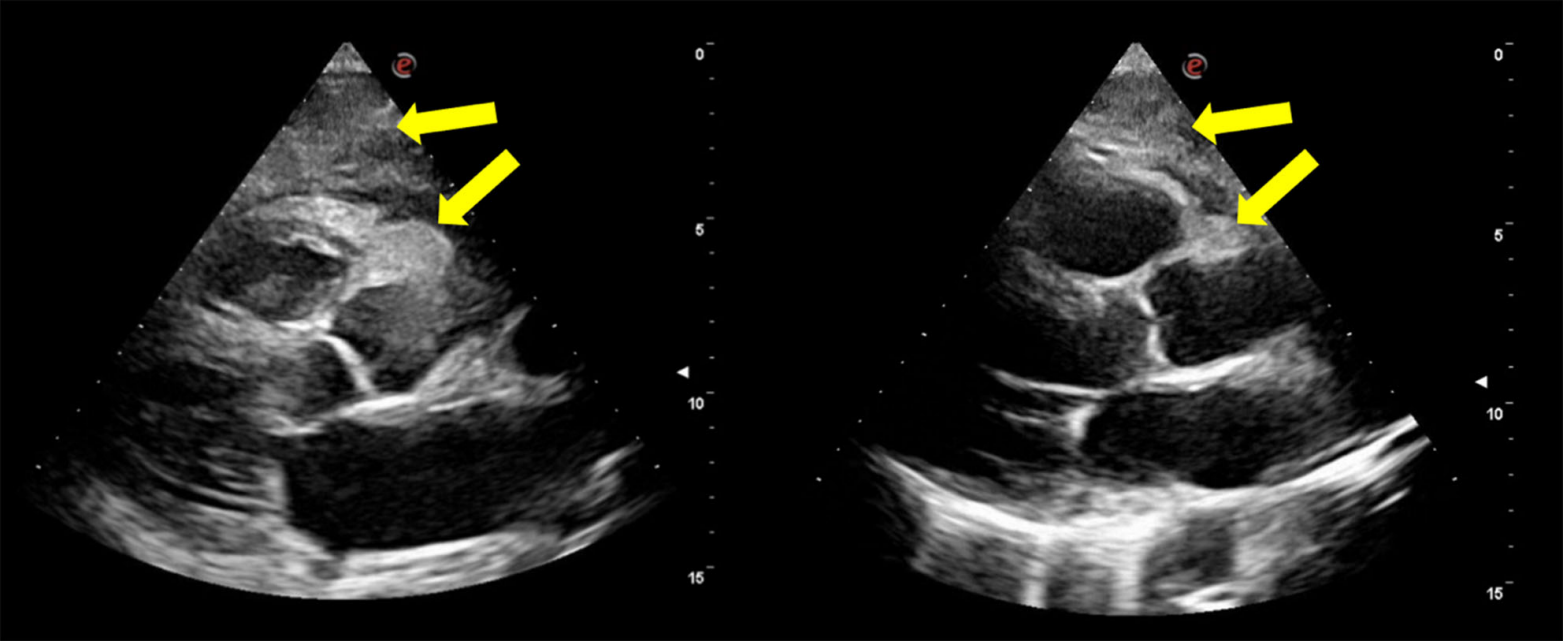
Trans-thoracic echocardiography - parasternal long-axis view. On the left, it is evident the marked and diffused thickening of the myocardium, especially of the interventricular septum, the posterior, the interatrial septum and the right ventricular free wall, which appear hypokinetic. Also, the large iso-echogenic mass takes up space around the ascending aorta and in the anterior mediastinum (yellow narrow). On the right, the view after the first chemotherapy regimen
